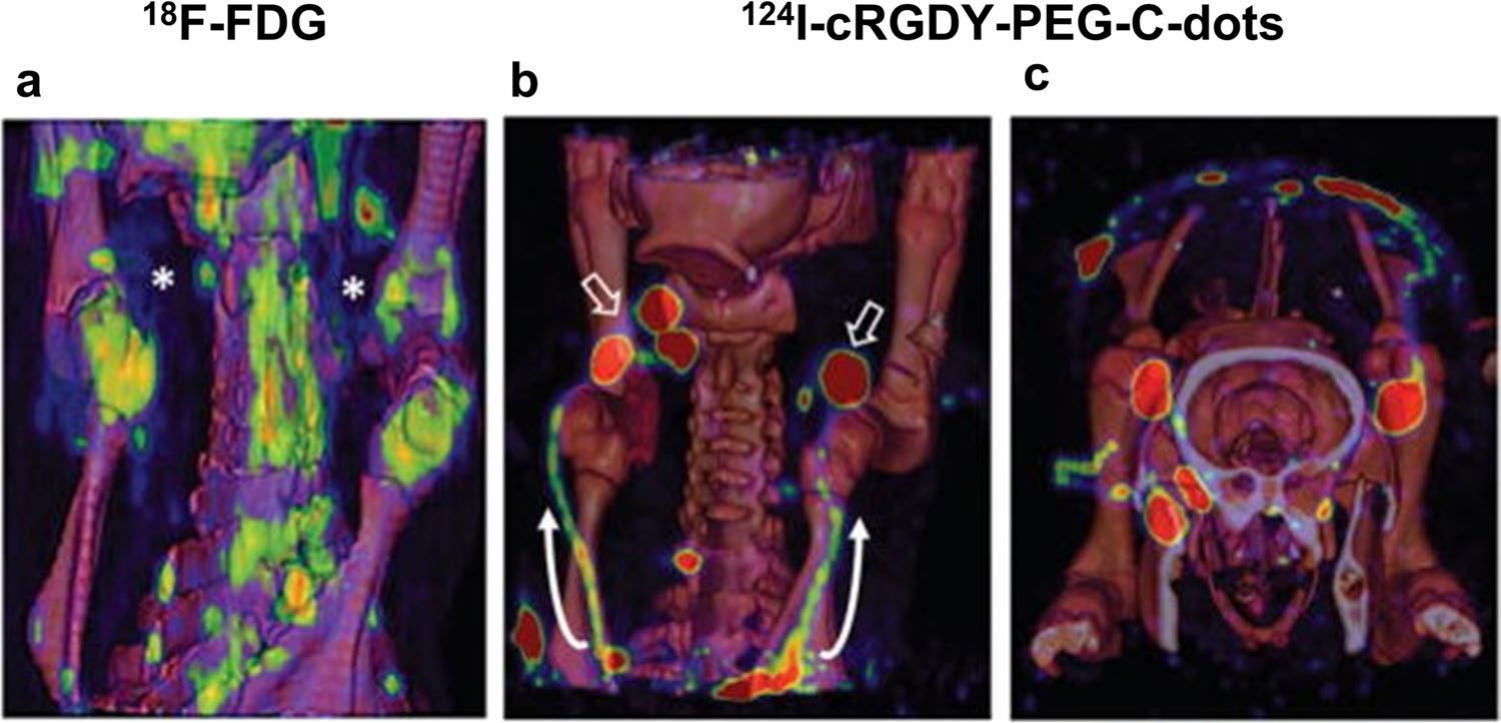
3D PET-CT fusion maximum intensity projection images integrated 18F-FDG and 124I-cRGDY-PEG-C dot PET-CT. a In the 18F-FDG PET-CT fusion image (coronal view) there is no evident nodal metastases (asterisks). Increased activity within bony structures is identified. b, c In contrast to the 18F-FDG image, the 124I-cRGDY-PEG-C-dot high-resolution PET-CT fusion images show coronal (b) and superior views (c) of bilateral metastatic nodes (open arrows) and lymphatic channels (curved arrows) within the neck following local injection of 124I-cRGDY-PEG-C dots. (Adapted with permission from Bradbury et al., 2013, ©2013, Integrative Biology, published by Oxford Academic)
